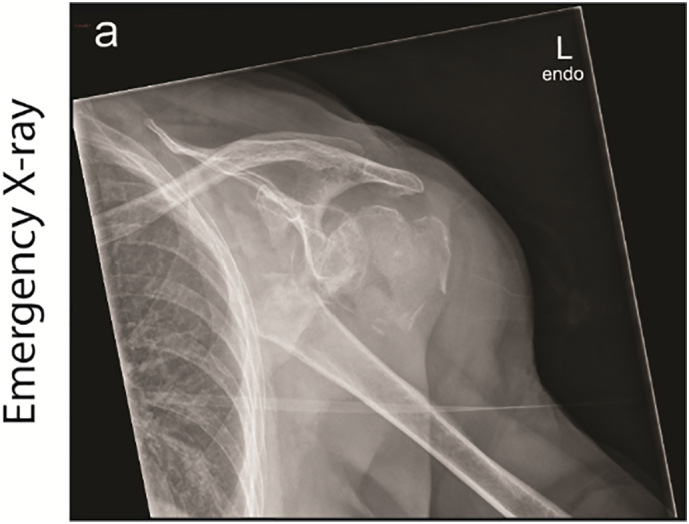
AP X-ray after trauma: Proximal humerus fracture with severe medial dislocation of the shaft, free greater tuberculum and multiple smaller osseous fragments.
